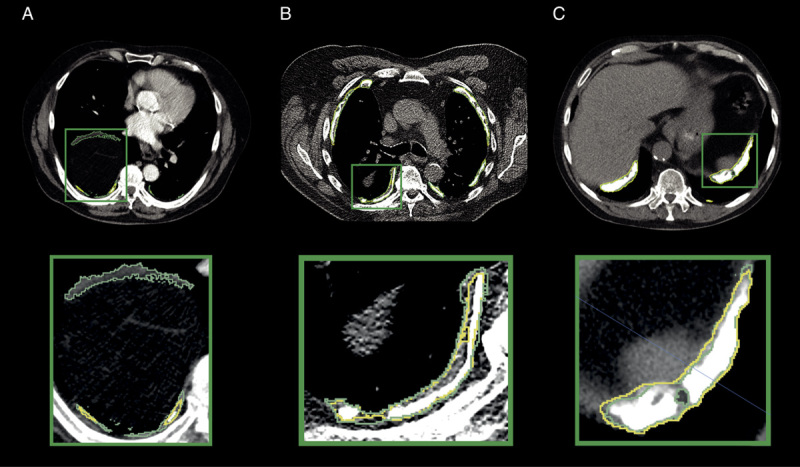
A, CT scans in the axial plane with contrast; an example of the lower value of DSC between AI in yellow outline, and radiologist in green outline (DSC = 0.43). B, CT scans in axial plane without contrast; average segmentation performance (DSC = 0.75). C, CT scans in axial plane without contrast; well-segmented plaques on the diaphragm (DSC = 0.85).
